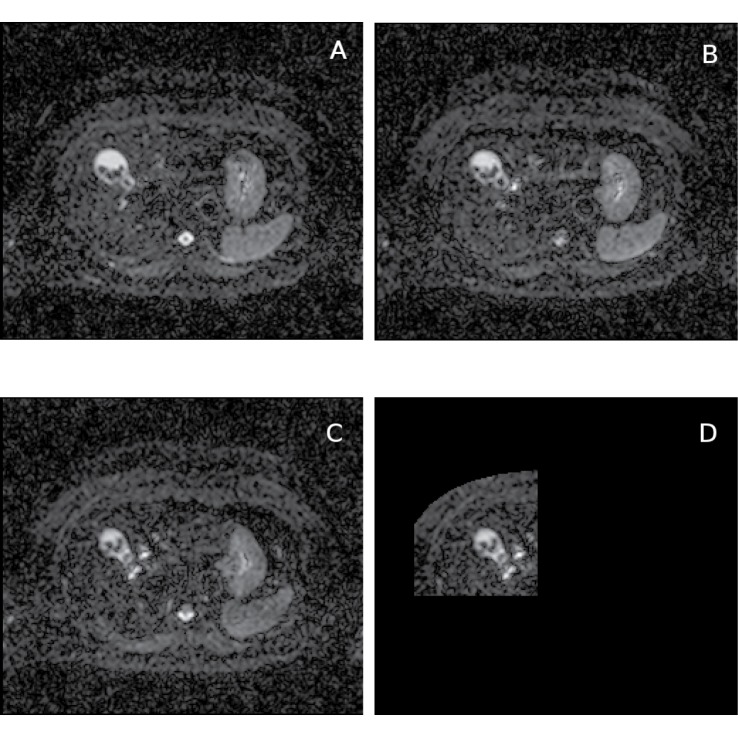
Diffusion gradient direction components of DW-MR data and the reference image. Healthy liver DW-MR image data (v3-0528-slc24); b-value = 100*s*/*mm*
